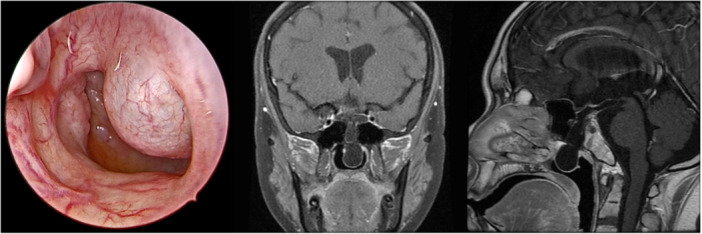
Endoscope and magnetic resonance imaging images reveal the meningoencephalocele protruding through sellar floor and sphenoid sinus into posterior nasal cavity.
